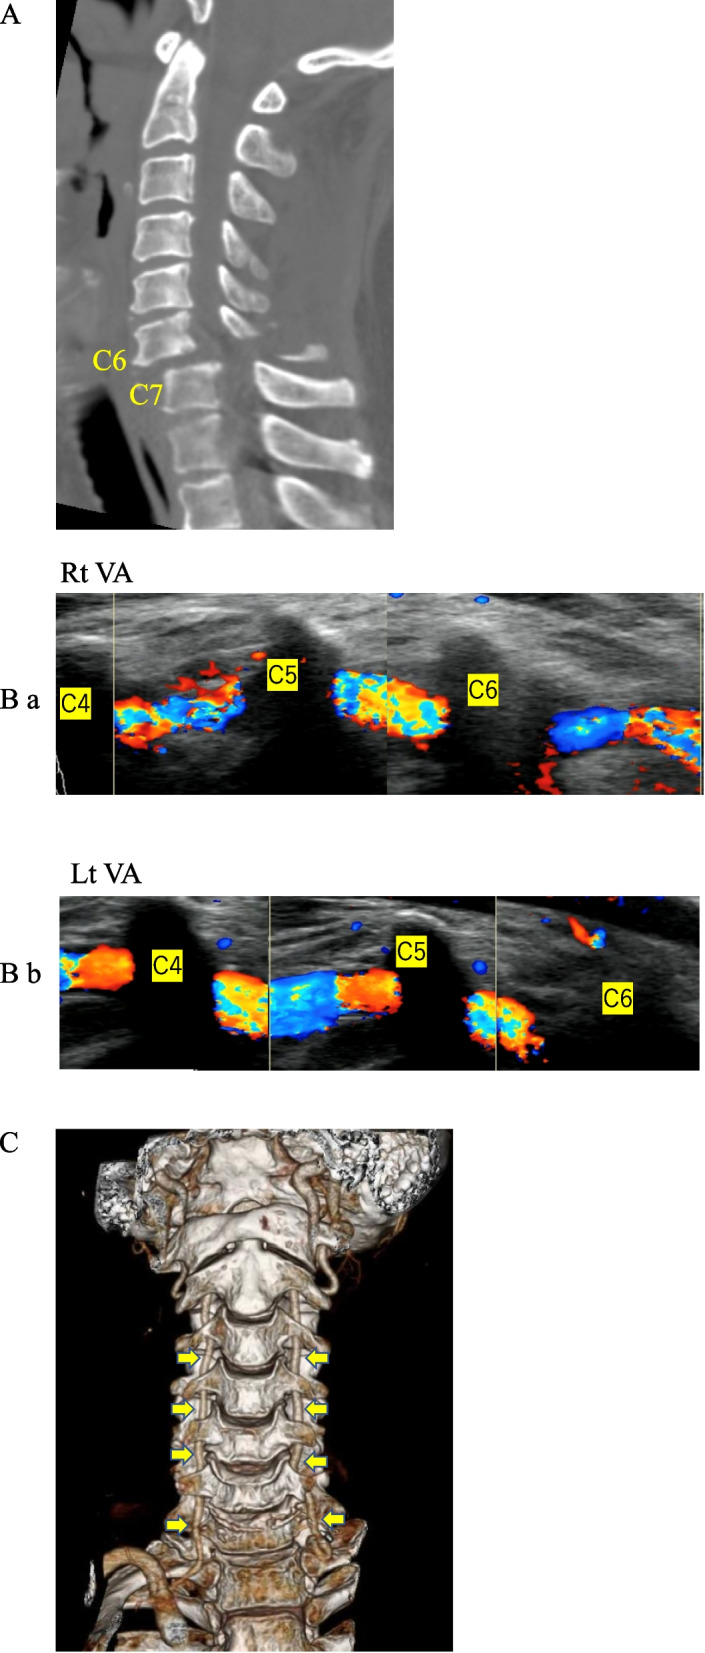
A CT shows the dislocation fracture at C6/C7. B ab. Ultrasonography shows blood flow in both VAs. C CT angiography shows blood flow in both VAs. (Arrow: VA)
